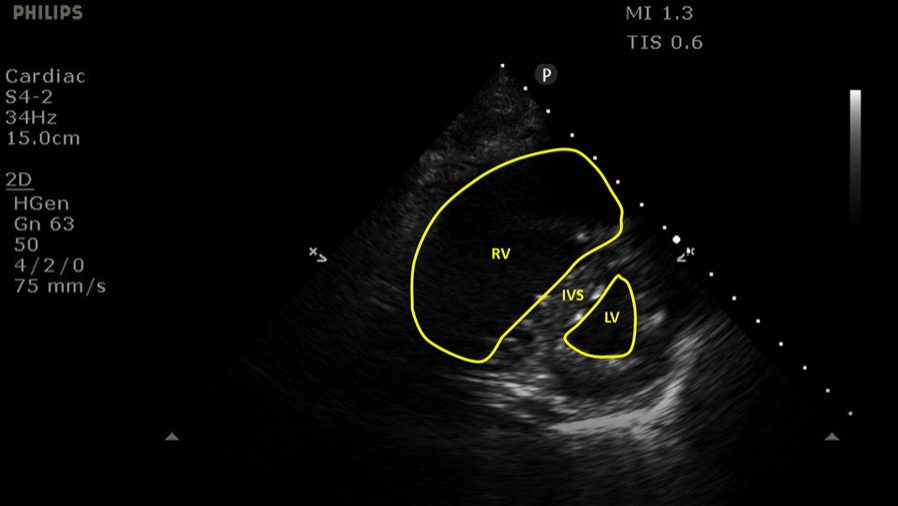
An example of acute cor pulmonale. 82-year-old woman with myelodysplastic syndrome was intubated for severe community-acquired pneumonia. She had no known cardiac problems or chronic lung disease. On admission to the intensive care unit, basic echocardiography was done. The parasternal short axis view showed a dilated right ventricle (RV) in end diastole at the papillary muscle level and interventricular septum (IVS) straightening, indicating acute cor pulmonale. The RV and left ventricle (LV) areas may be determined via endocardial tracing as shown, though in many cases, an RV/LV ratio of ≥ 1 can be determined visually without routine manual tracing
